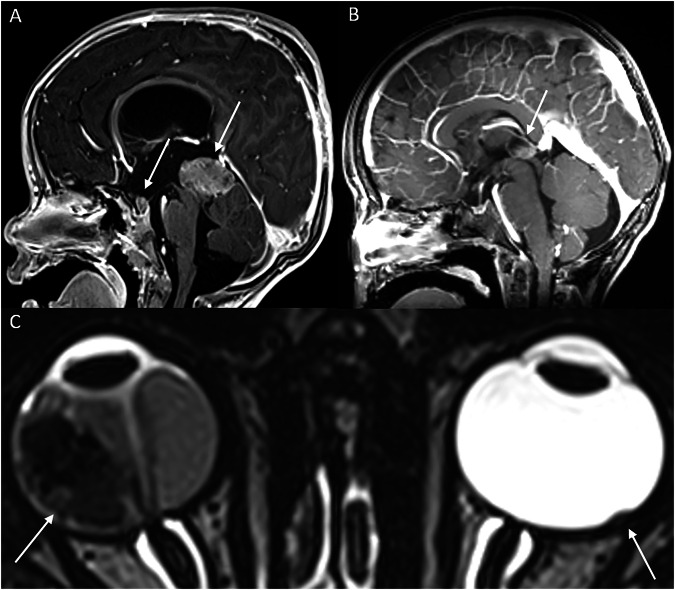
Midline embryonal tumors and trilateral retinoblastoma: A Sagittal contrast-enhanced T1-weighted image of a 2-year-old boy with a history of bilateral retinoblastoma since birth, showing midline embryonal tumors in both the suprasellar and the pineal regions (arrows). B Sagittal contrast-enhanced T1-weighted image demonstrating a pinealoblastoma (arrow). C Axial heavily T2-weighted image from the same patient revealing bilateral retinoblastoma (arrows)
